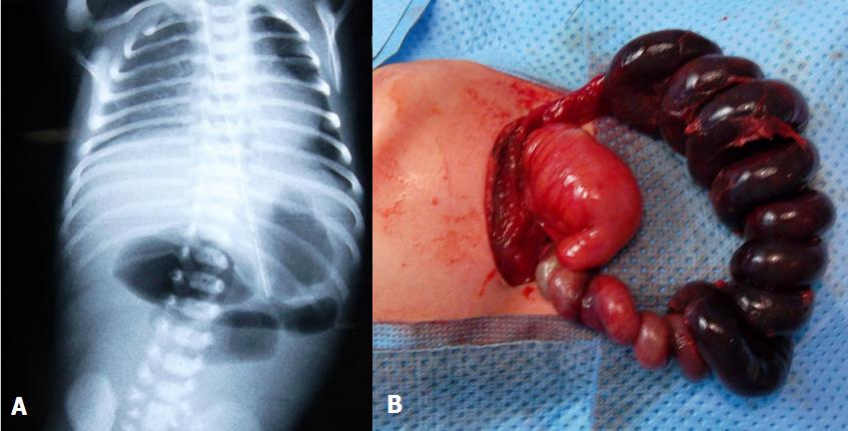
(A) Radiographie thoraco- abdominale sans préparation montrant des niveaux hydro-aériques de type grêlique; (B): aspect per-opératoire objectivant une atrésie grêlique en queue de cochon avec nécrose de la presque totalité des anses intestinales
